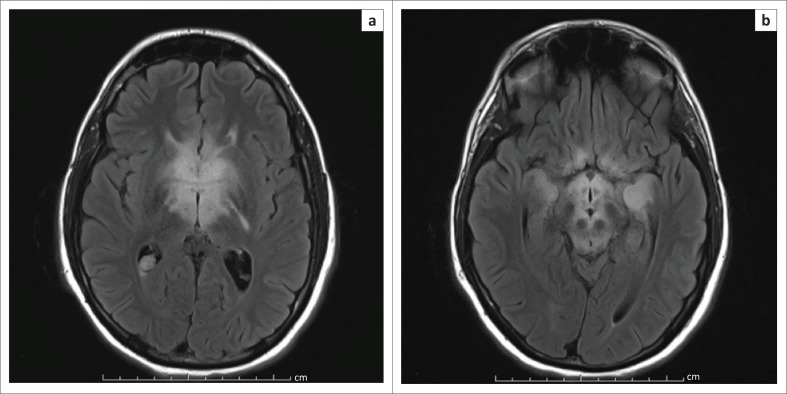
(a) Axial Fluid Attenuation Inversion Recovery (FLAIR) imaging demonstrating symmetric increased signal involving the basal ganglia and thalami bilaterally, (b) Increased signal intensity is also noted in the hypothalamus, anterior midbrain and to varying degrees in both medial temporal lobes.
